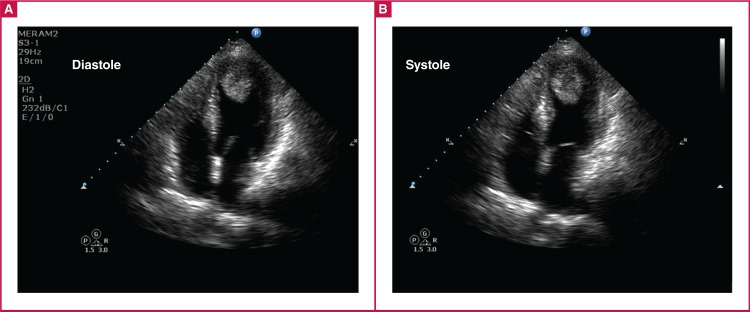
Initial echocardiography showing apical ballooning and apical thrombus in the diastolic and systolic phase.
